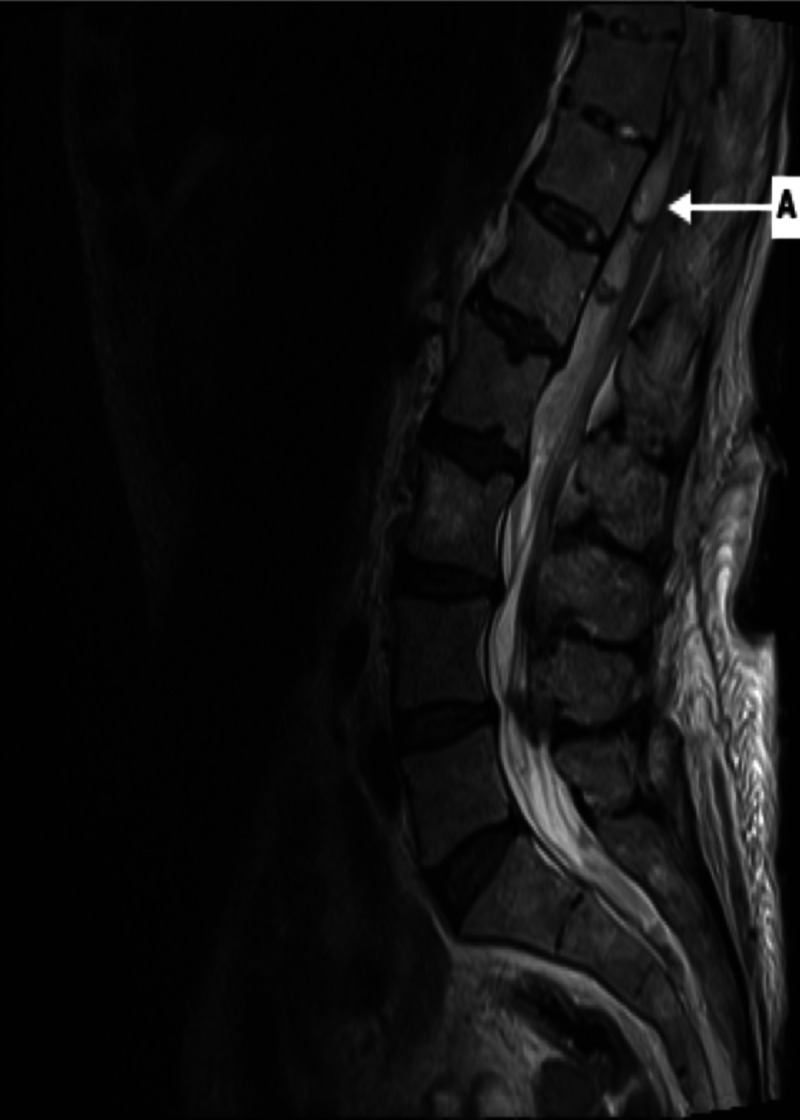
Magnetic resonance imaging T2 sagittal view Arrow A points to a heterogeneous collection at the anterior epidural space at T12 and L1 measuring 4.9 cm in length
